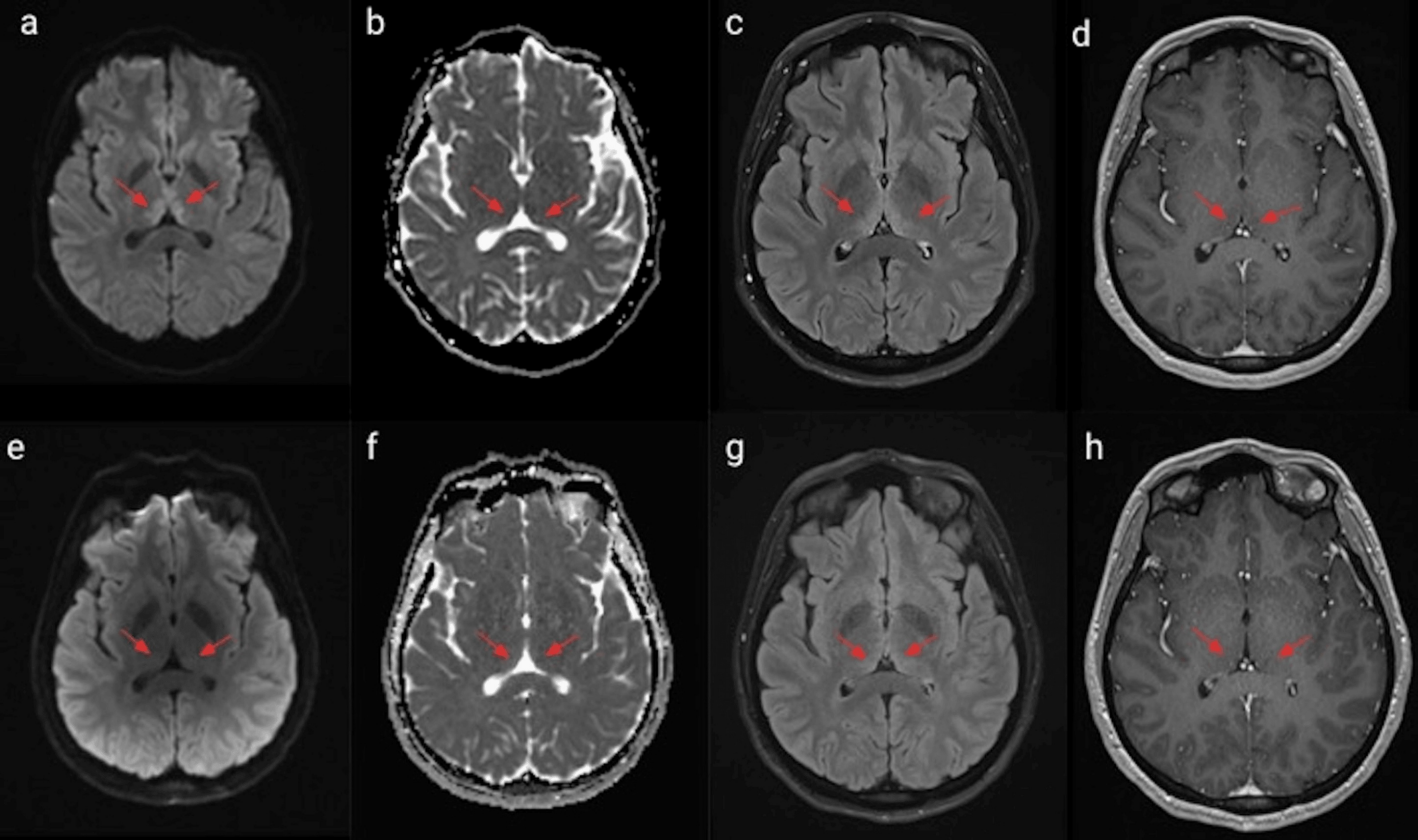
MRI brain comparison of thalami/pulvinar on admission and four months later MRI brain comparison of thalami/pulvinar on admission (the top row) and four months later (the bottom row): diffusion restriction (a, b) and hyperintensity in T2-weighted-Fluid-Attenuated Inversion Recovery (T2 FLAIR) sequence(c). Resolving signal abnormalities with no diffusion restriction (e, f) and minimal residual elevated T2/FLAIR signal (g) in the medial aspect of the thalami, T1-weighted images are normal (d, h).
